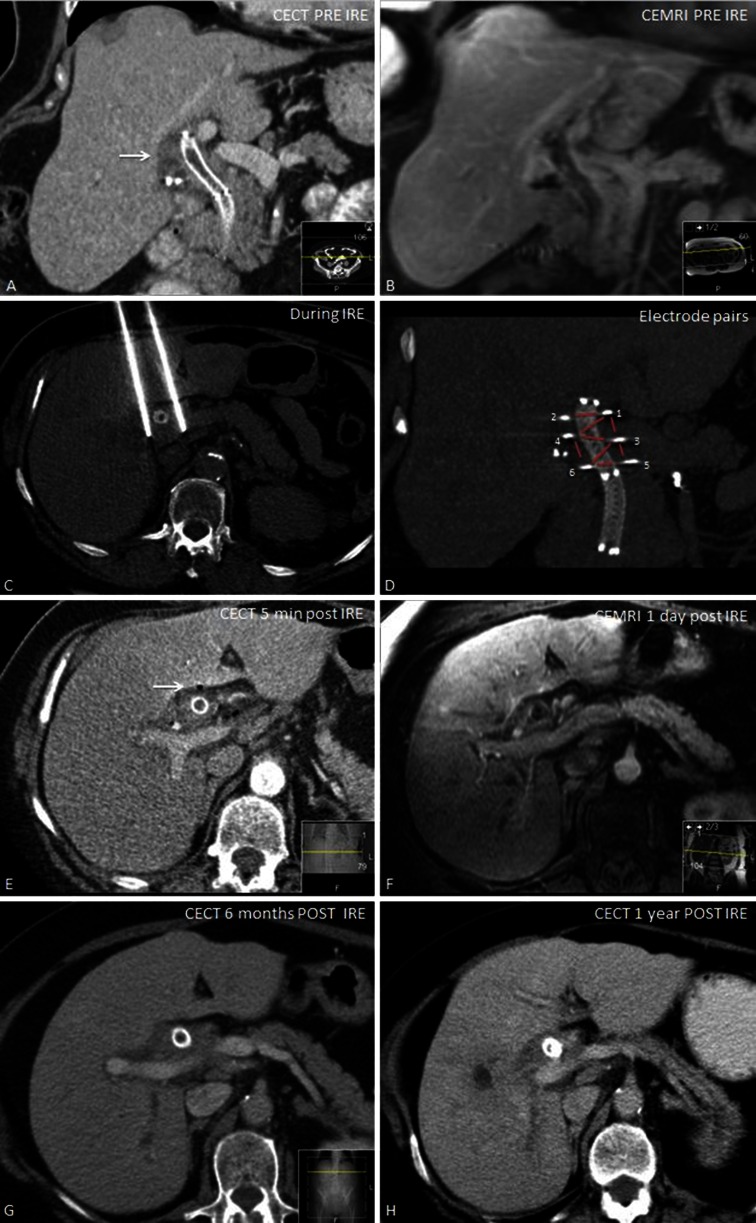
A Coronal ceCT image pre IRE with a hilar cholangiocarcinoma (*arrow*) surrounding a metallic Wallstent present in the common bile duct. B ceMRI image demonstrating an enhancing mass in the liver hilum surrounding the common bile duct. C Axial CT image of two electrodes placed alongside the Wallstent. D Coronal CT view demonstrating all six electrodes and eight electrode pairs during the ablation (*red lines*). E ceCT immediately after IRE demonstrating patent vessels and gas bubbles in the ablated area (*arrow*). F ceMRI 1 day post-IRE with no signs of complications. G, H ceCT 6 months and 1 year after IRE demonstrating no tumor progression
